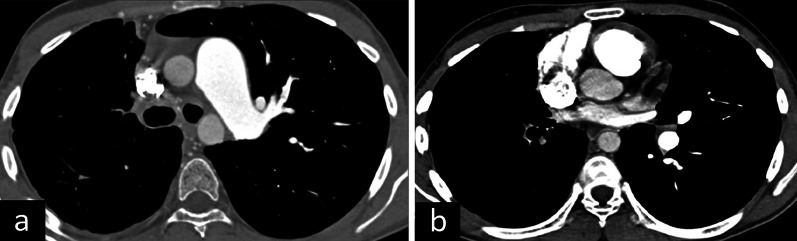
A 20-year-old man with proximal interruption of the pulmonary artery (PIPA). Contrast-enhanced axial CT images (a, b) show the lack of the proximal portion of the right main pulmonary artery
